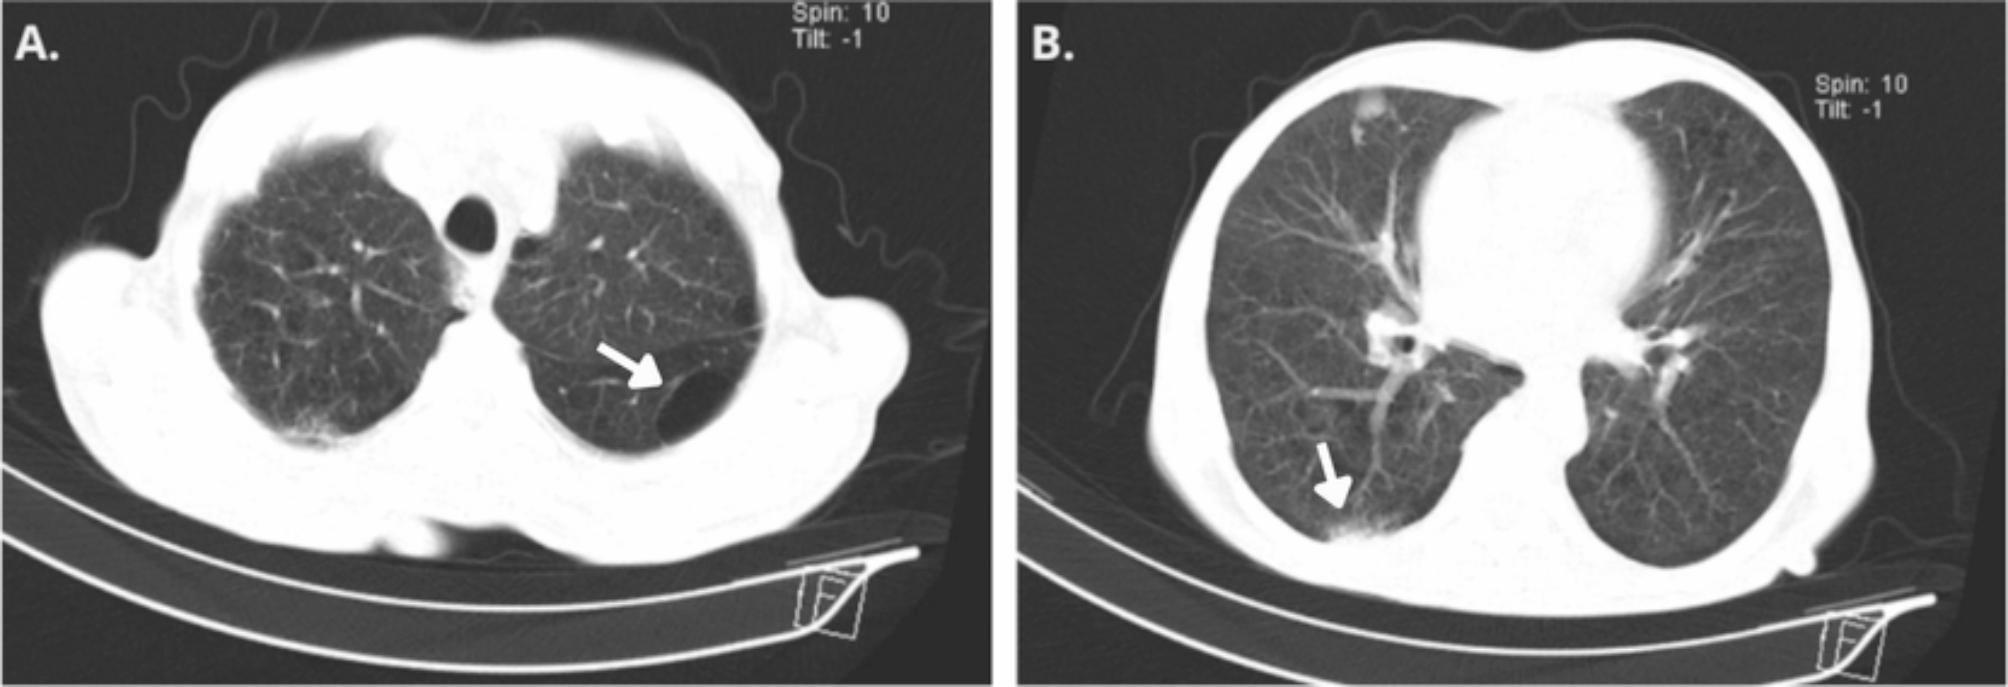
High-resolution chest computed tomography scan. (A) Subpleural bulla measuring 5 × 2.5 cm in the apical segment of the left lower lobe. (B) Diffuse hypodensity of the lung parenchyma, with hypodense wall images predominantly in the lower lobes
